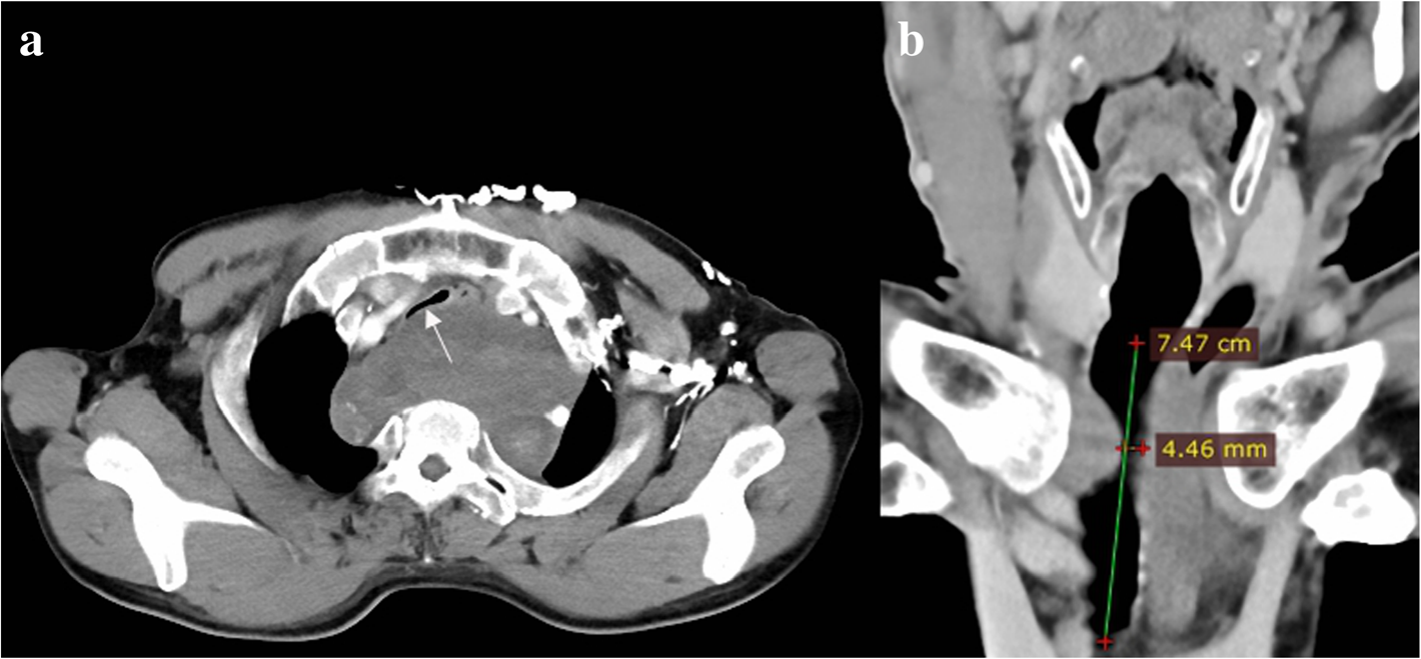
The preoperative CT image of the narrowest inner diameter of malactic trachea (a transverse section, b coronal section)
